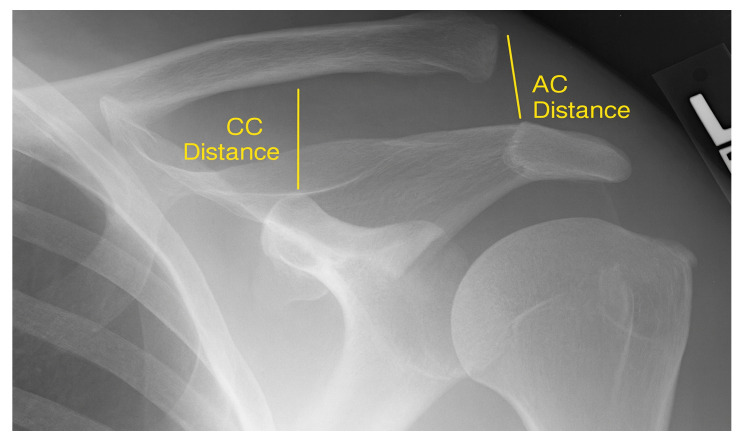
Anteroposterior radiograph of the shoulder demonstrating measurement of the acromioclavicular (AC) distance and the coracoclavicular (CC) distance.
